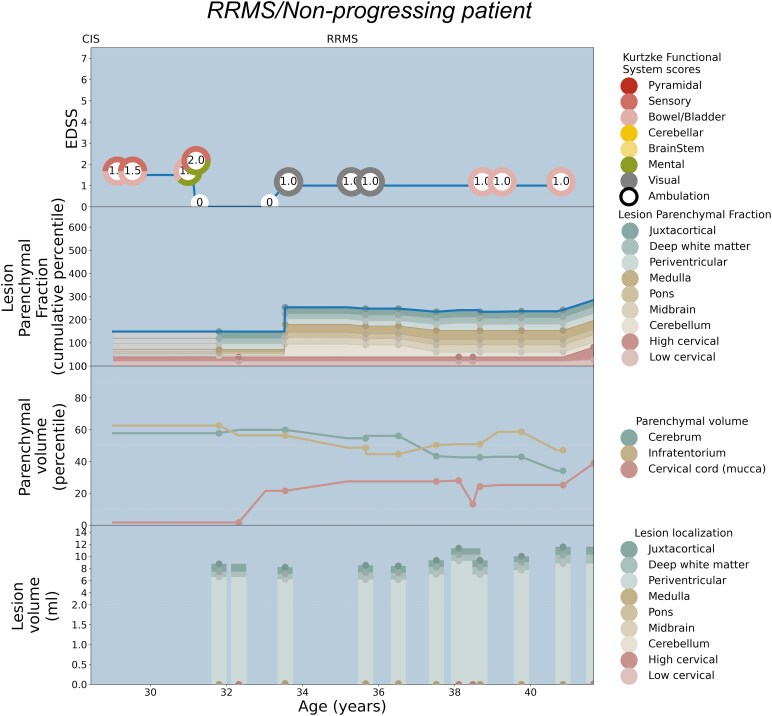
Patient depiction demonstrating stable RRMS without conversion to SPMS, and the corresponding LPF trajectories aligning with minimal symptoms. See the legend of Fig. 3 for a general explanation of the figure panels. The patient maintains mild disability between the ages of 28 and 42, with a period of time with an EDSS of 0 in her early 30s, and does not convert to an SPMS phenotype. Although she demonstrates a relatively large total lesion load (bottom panel), the lesions are mostly located in the cerebral hemispheres, which contribute least in this model to the overall EDSS score. The lesion volume in the spinal cord and infratentorial compartment is minimal, yielding a largely flat cumulative LPF profile consistent with the low disability curve. At several time points in her mid-30s, the patient’s EDSS score is 1.0 based on visual findings alone, shown in grey in the colour wheel as there is no MRI correlate for this functional system.
